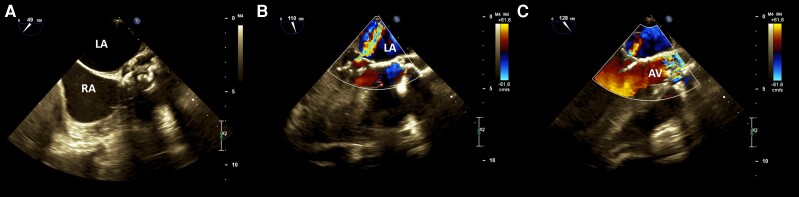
Trans-oesophageal echocardiography in short and long-axis views showed calcified aortic valve’s cusps with the extension of non-coronary cusp’s calcification to the anterior mitral valve leaflet, resulting in some degree of mitral stenosis (*A* and *B*). The same as the trans-thoracic echocardiography, again we can prove the severe regurgitation of blood through the mitral valve and significant increase in velocity of blood while passes through the aortic valve (*C* and *D*). AV, aortic valve; LA, left atrium; RA, right atrium.
